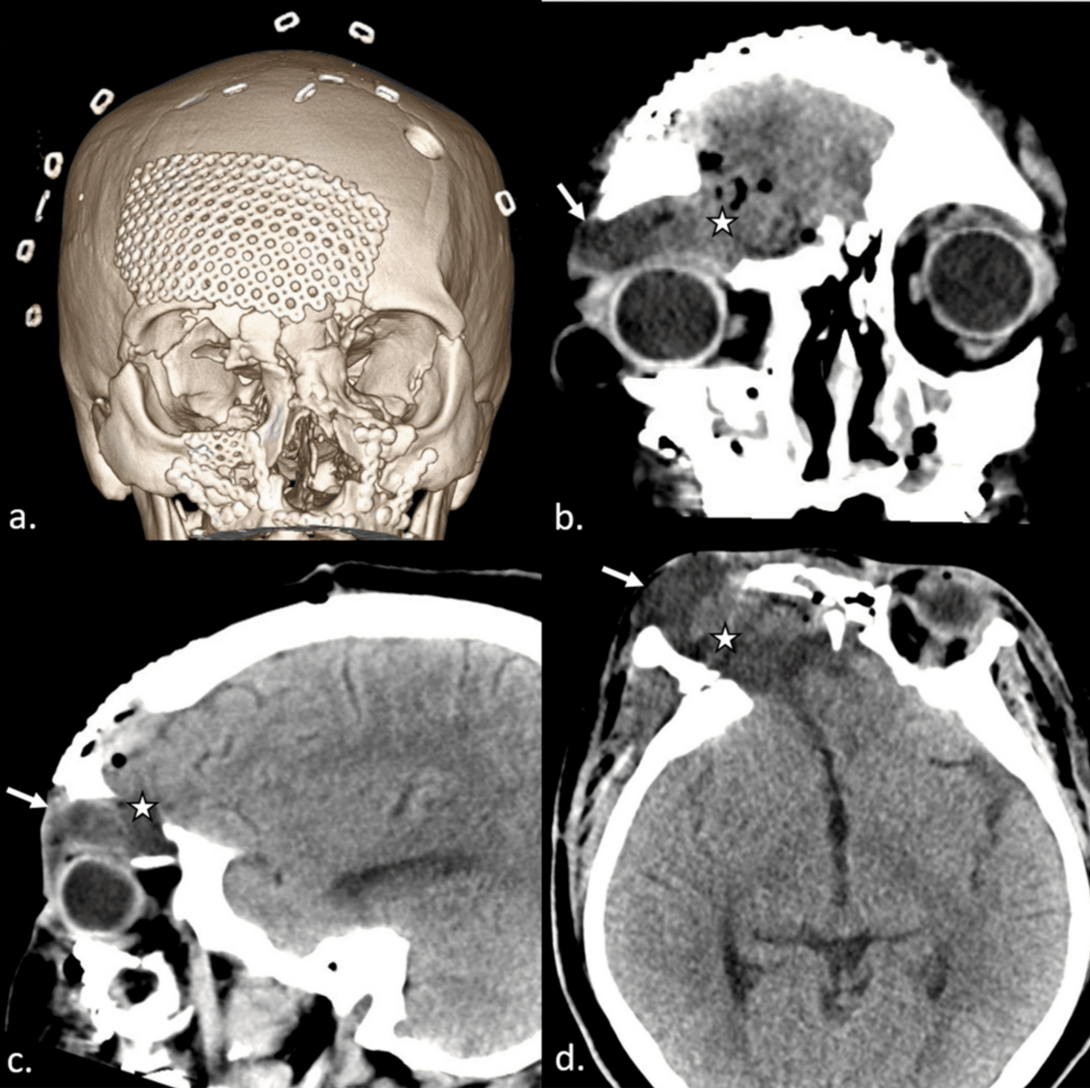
Further follow-up CT The 3D volume-rendered image (a) shows postsurgical changes after the revision of frontal cranioplasty with mesh placement to address the upper forehead aspect of the pseudomeningocele with a resolution of the pseudomeningocele along the forehead. Coronal, sagittal, and axial plane images (b, c, and d) demonstrate persistence, but the decreased size of the pseudomeningocele extending into the right superior orbit and periorbital space (white arrows) through a persistent defect in the right orbital roof (white stars). CT: computed tomography, 3D: three dimensional
